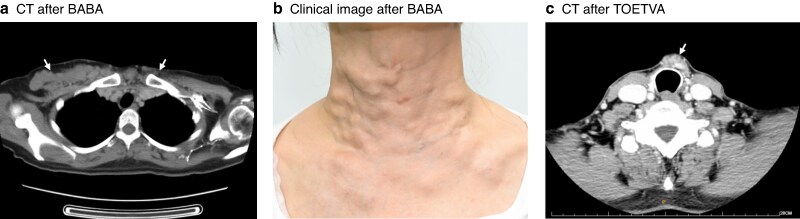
Representative images showing recurrence and tumour seeding after BABA and TOETVA requiring reoperation a, b Patient after BABA. The initial thyroidectomy pathology was follicular adenoma, with reoperation revealing follicular thyroid carcinoma. a Contrast-enhanced CT showing multiple recurrent lesions (arrows) in the anterior neck and chest within the previously dissected flap. b Clinical photograph showing multiple recurrences on the patient’s neck and anterior chest, necessitating wide dissection and additional skin incisions. c Patient after TOETVA. The initial pathology was follicular adenoma, and reoperative pathology confirmed follicular thyroid carcinoma. Contrast-enhanced CT demonstrated a suspicious recurrent lesion (arrow) in the suprastrap muscle area of the anterior neck within the previously dissected flap. BABA, bilateral axillo-breast approach; TOETVA, transoral endoscopic thyroidectomy vestibular approach; CT, computed tomography.
